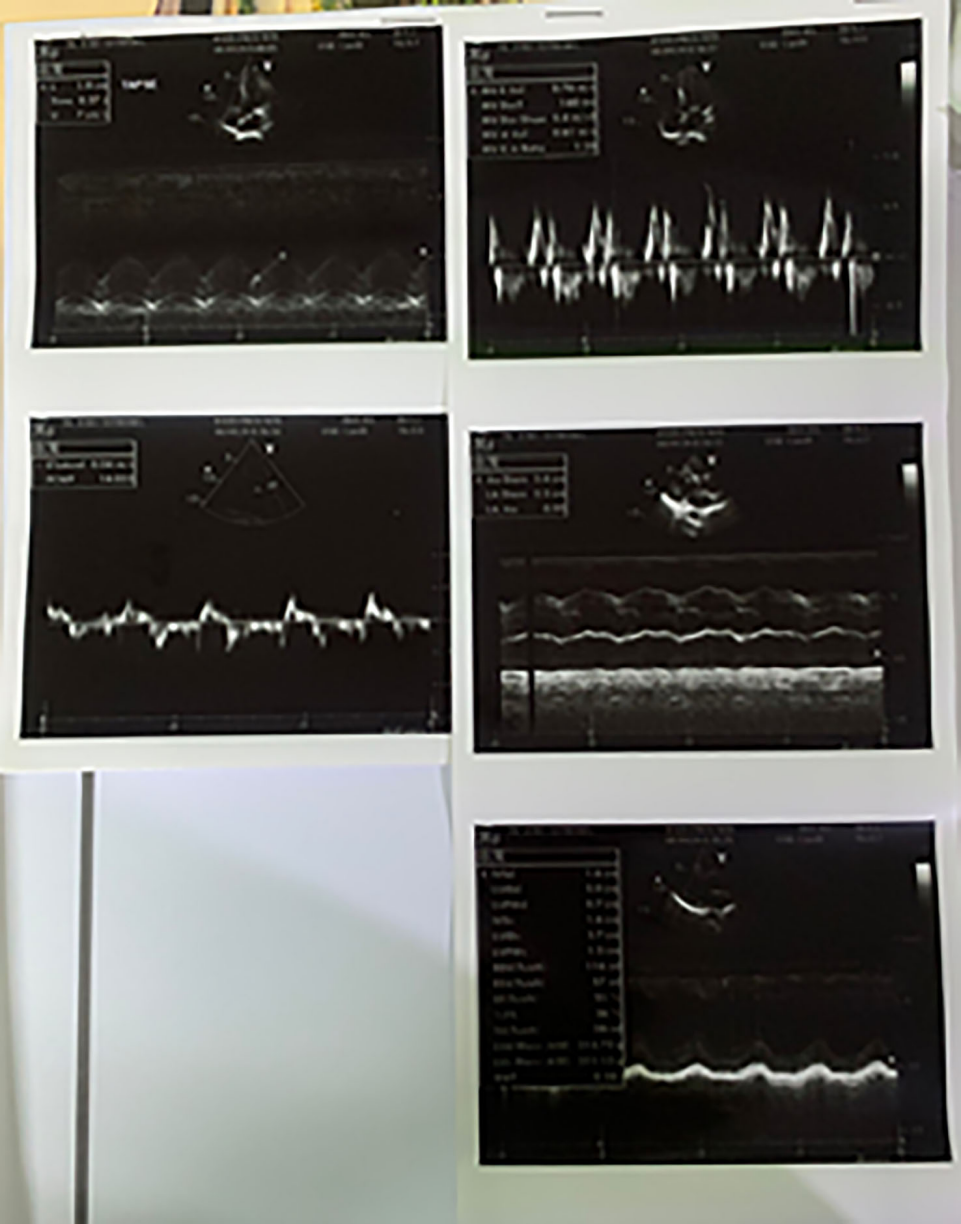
Patient's echocardiography after primary PCI.
